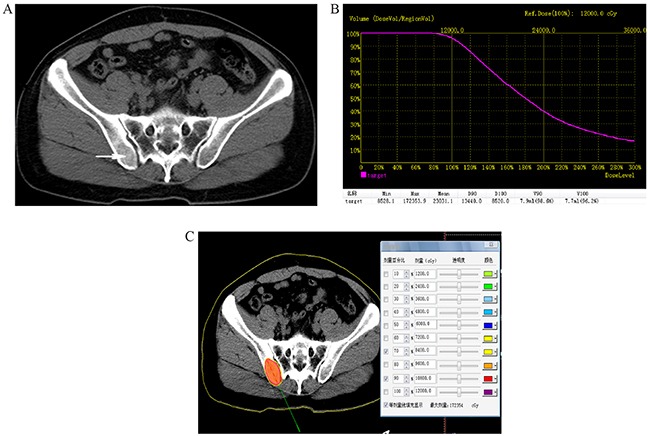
A. Preoperative computed tomography (CT) image was obtained for targeting bone areas of interest, which showed one lesion in right iliac bone (arrowhead). B. Dose volume histograms (DVH). The prescription dose is 120 Gy during the planning. A total of 90% of the tumor target (D90) received 134.4 Gy, and 96.2% of the tumor received 100% of the prescribed dose (V100 = 96.2%). C. Isodose curves plotted by the treatment planning system (TPS), the planning target volume (PTV) edge was covered by isodose curve from 70% to 90%.
